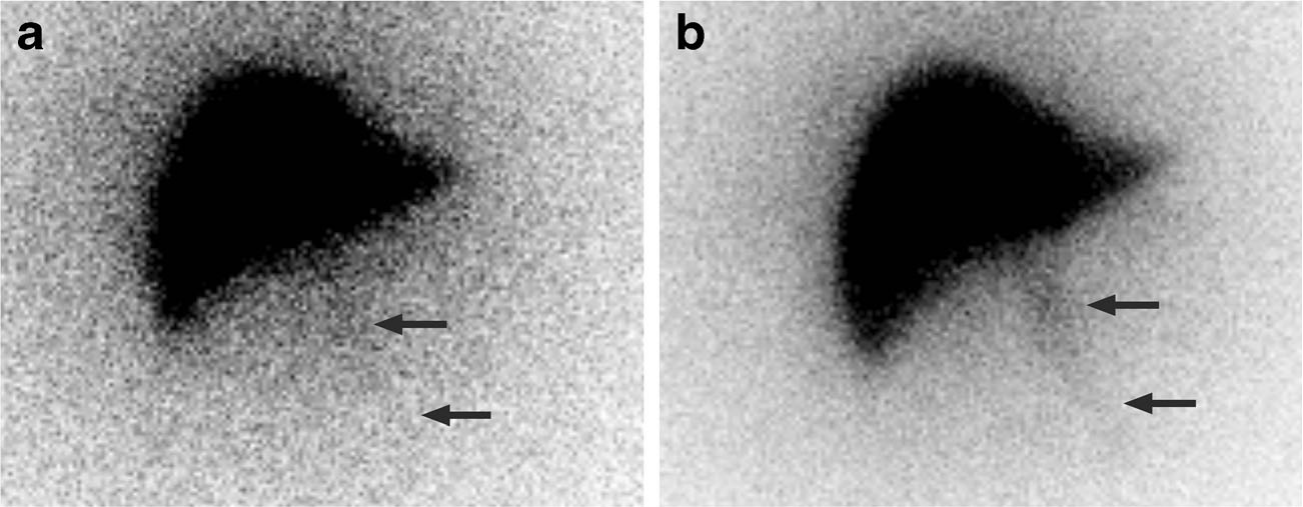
A 60-year-old male with colorectal liver metastases. (a) Planar ^166^Ho image of scout dose, depicting a faint extrahepatic deposition in falciform ligament. (b) Post-treatment planar ^166^Ho image, depicting similar extrahepatic deposition in falciform ligament (SPECT/CT images not shown)
